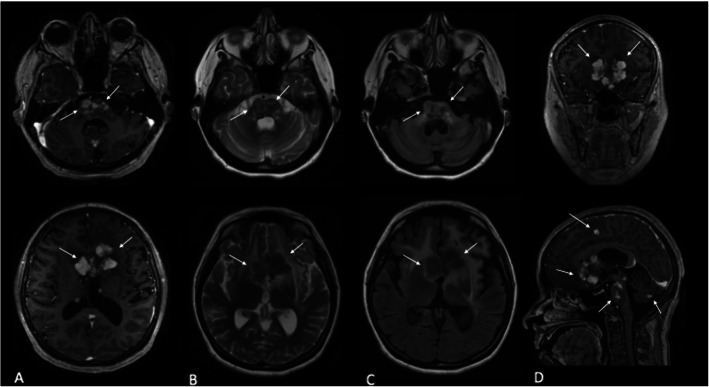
Brain MRI showing bilateral enhancing intraparenchymal mass lesions, most of them located in the pons and anterior basal ganglia (white arrows), surrounded by massive vasogenic edema and predominantly with low signal on T2 weighted images. a Axial T1 post contrast at the level of pons and basal ganglia. b Axial T2 images. c Axial FLAIR images. d Coronal (on top) and sagittal T1 (bottom) post contrast images. Note that the lesions sometimes follow perivascular spaces topographies
